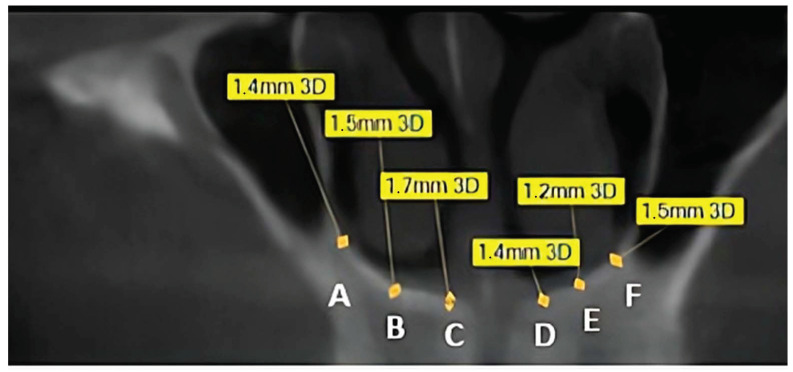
Cone beam computed tomography (CBCT) scan of the maxilla in coronal view with measurement points labeled (A–F). Yellow boxes indicate cortical bone thickness values (mm). See Table 1 for anatomical definitions of each point.
